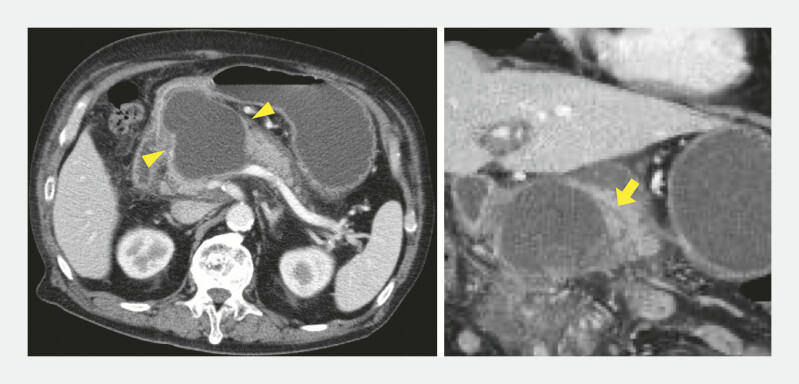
A computed tomographic image of the pancreatic pseudocyst (arrowhead) and the slightly dilated MPD (arrow). MPD, main pancreatic duct.
